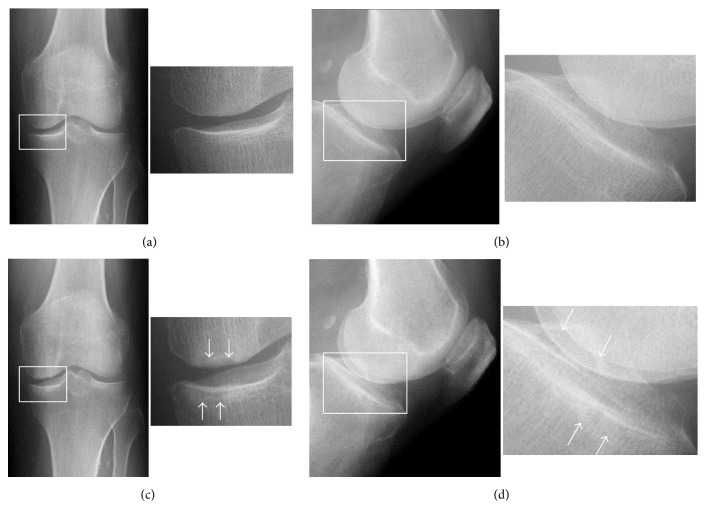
Plain initial (a) frontal and (b) lateral radiographs, showing slight subchondral bone sclerosis on the tibial medial condyle, but no evidence of radiolucent lesions or joint space narrowing. (c) A frontal radiograph and (d) its enlarged view, taken 3 months after the first evaluation, showing a radiolucent oval shadow in the subchondral areas of the weight-bearing portions of both the MFC and MTP, surrounded by a sclerotic halo (arrow).
